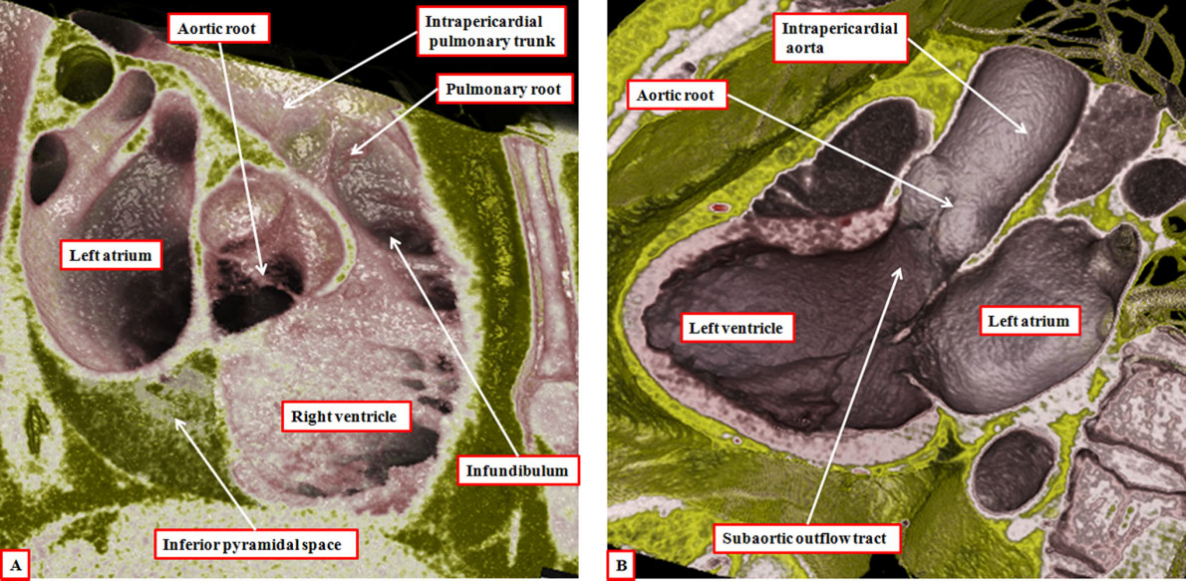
The virtual dissections are made from a data set obtained using multidetector computed tomography in a patient undergoing investigation for coronary arterial disease. They show how each outflow tract is formed in a tripartite fashion, with the components represented by the intrapericardial arterial trunk, the arterial root, and the subvalvar outflow tract, respectively. Note that, in the right ventricle (panel A), the subvalvar component is a completely muscular infundibular sleeve, whereas in the left ventricle (panel B), the posterior wall of the subvalvar area is formed by fibrous continuity between the leaflets of the aortic and mitral valves.
